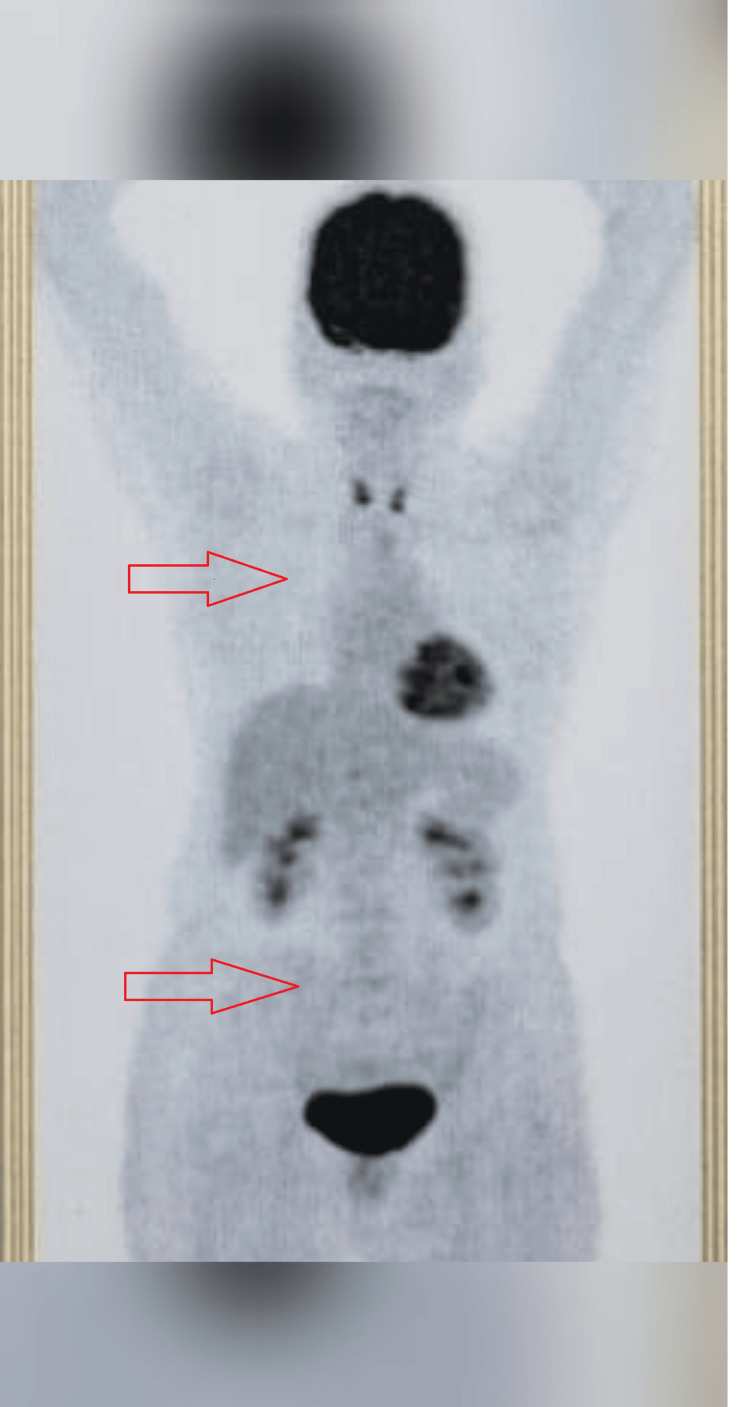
Post-chemotherapy positron emission tomography-computed tomography scan showing complete metabolic response.
